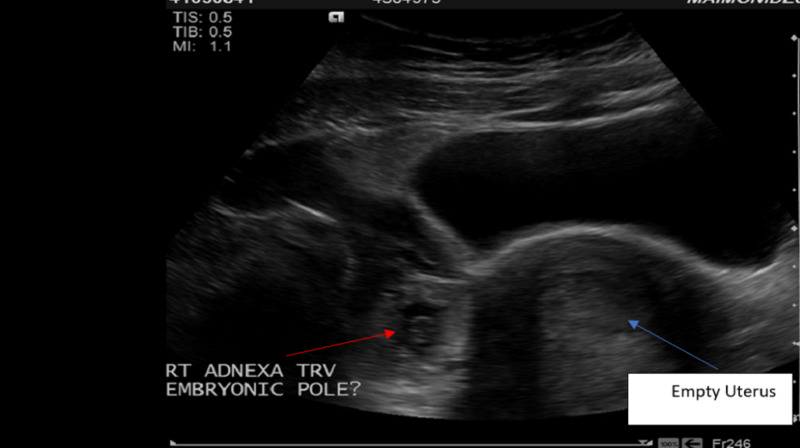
Pelvic ultrasound (transverse view): embryonic pole in the gestational sac in the right adnexal area
